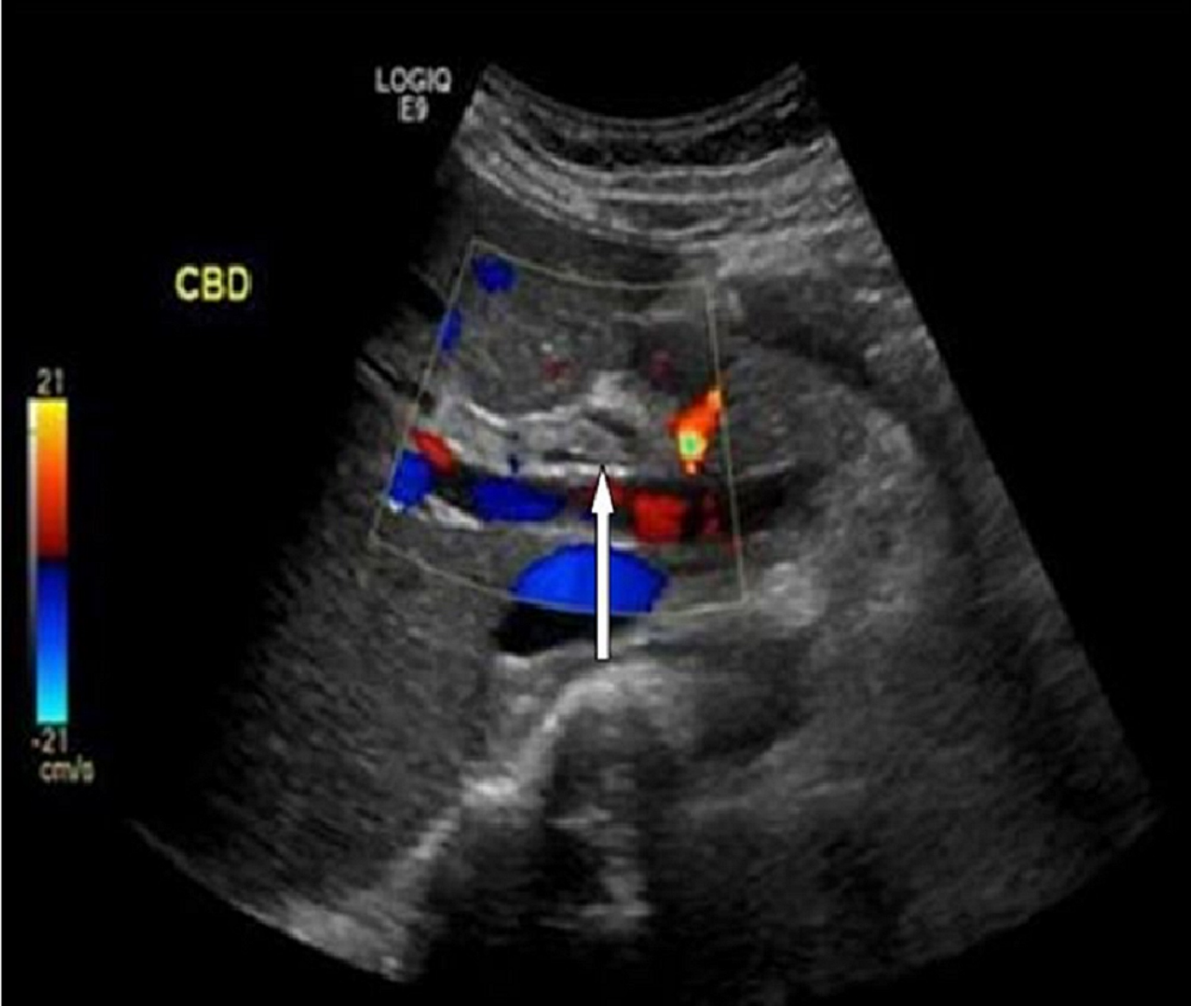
Biliary ultrasound depicting the radiological four-line sign (arrow)
